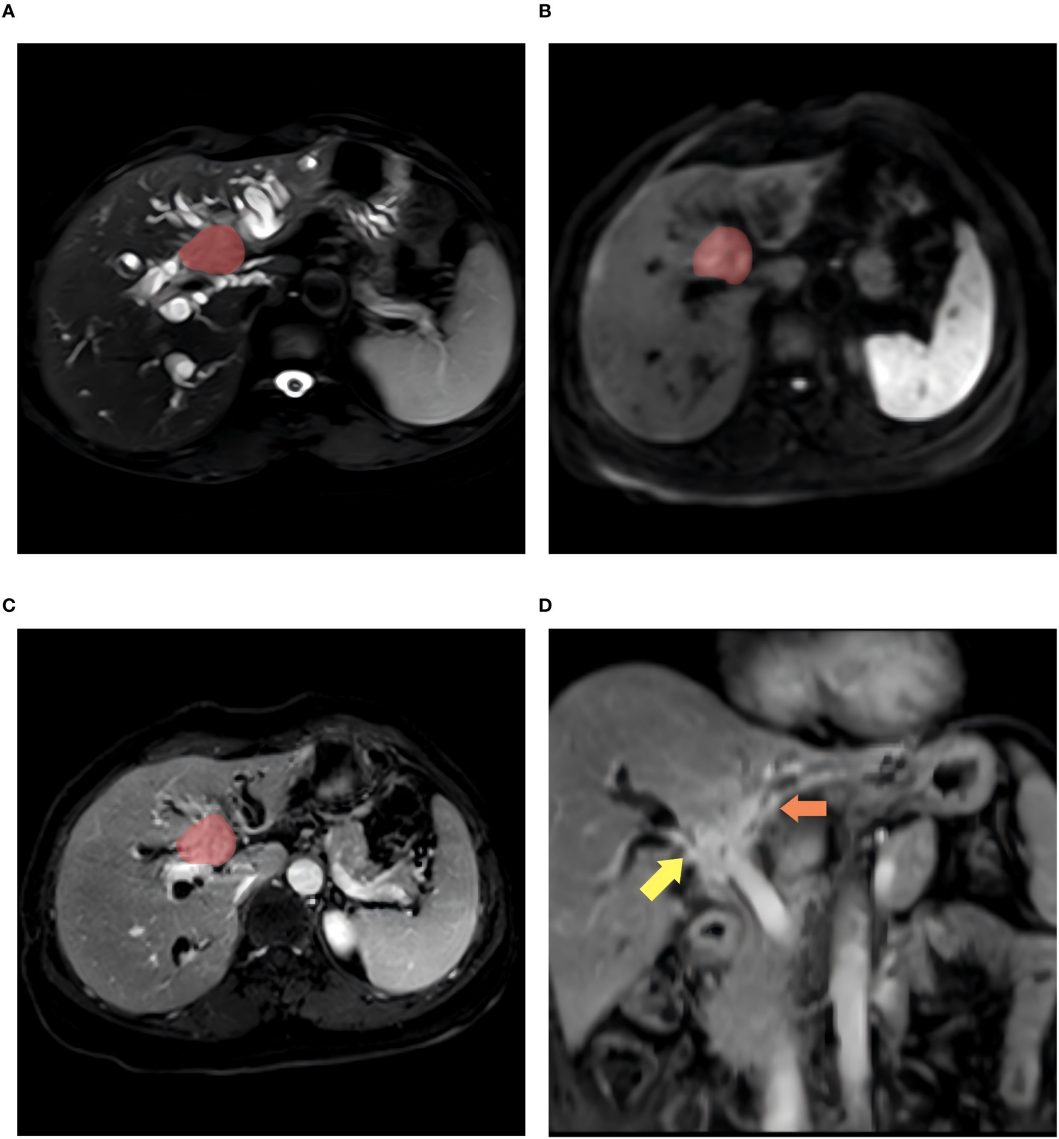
A 56-year-old woman with Bismush type IV HCCA. (A–C) shows the patient’s axial T2WI, DWI, and enhanced ROI contoured images, respectively, and (D) images show the affected areas at the bifurcation of the right hepatic duct and the left hepatic duct (indicated by the yellow arrows and the orange arrows).
